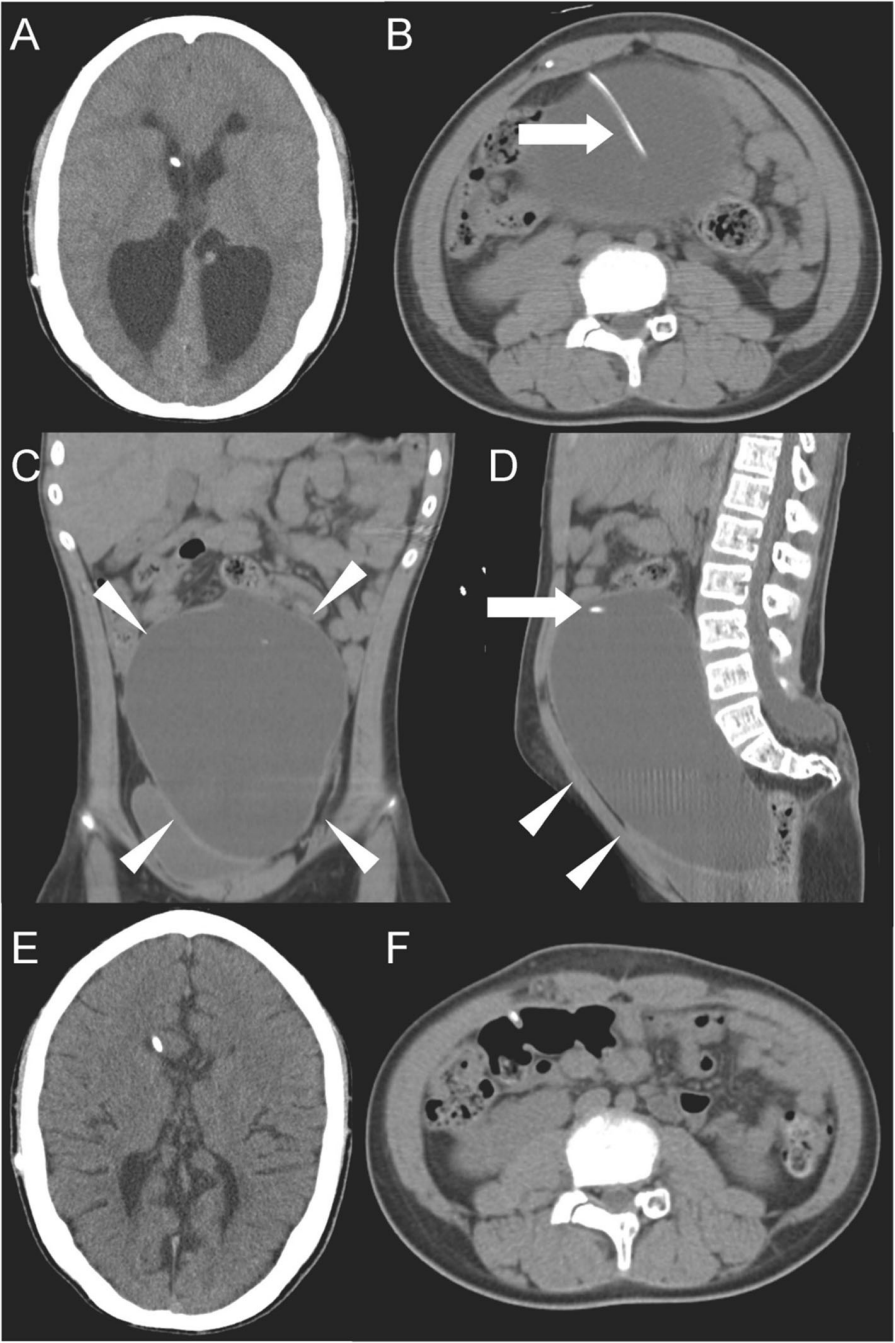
Cranial computed tomography demonstrated bilateral ventricular dilatation, periventricular edema, and effacement of sulci (a). Abdominal computed tomography demonstrated a cyst comprising a collection of homogeneous low-density fluid in the abdominal cavity with displacement of the bowel loops to the posterior part. The ventriculoperitoneal shunt catheter tip was located adjacent to the collection of fluid (b axial, c coronal, d sagittal). Computed tomography obtained before revision of the distal side of the peritoneal shunt catheter indicated improvement of the hydrocephalus (e). Computed tomography obtained after revision of the distal side of the peritoneal shunt catheter indicated no recurrence of the pseudocyst (f). The cyst and the shunt catheter are indicated by *white arrowheads* and *arrows*, respectively
